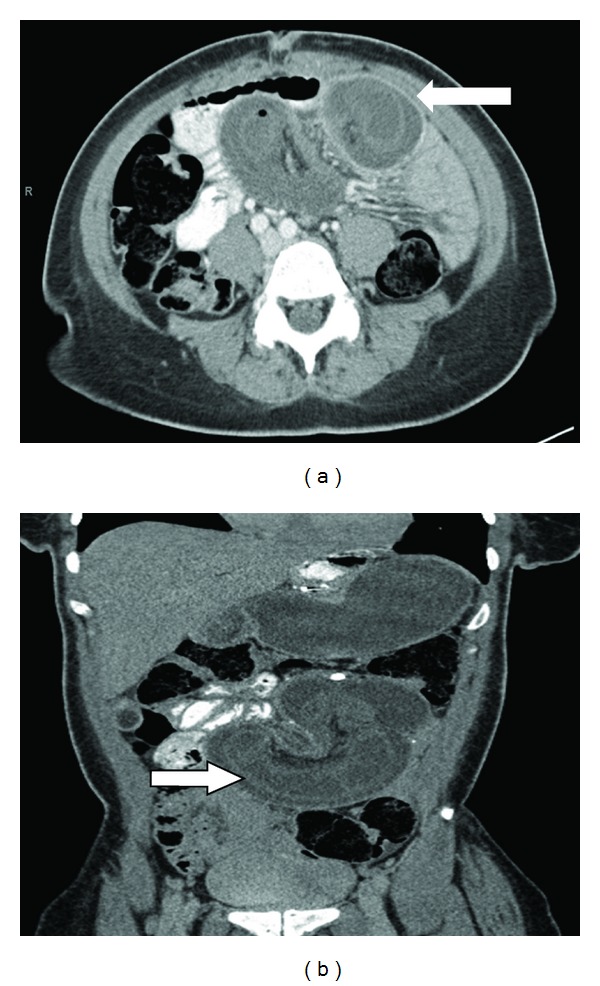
(a) Axial view of the CT scan showing intussusception with fat and blood vessels within the lumen of intestine (white arrow—target sign and pneumatosis). (b) Coronal view of the CT scan showing intussusception (white arrow—sausage-shaped thickened bowel wall).
